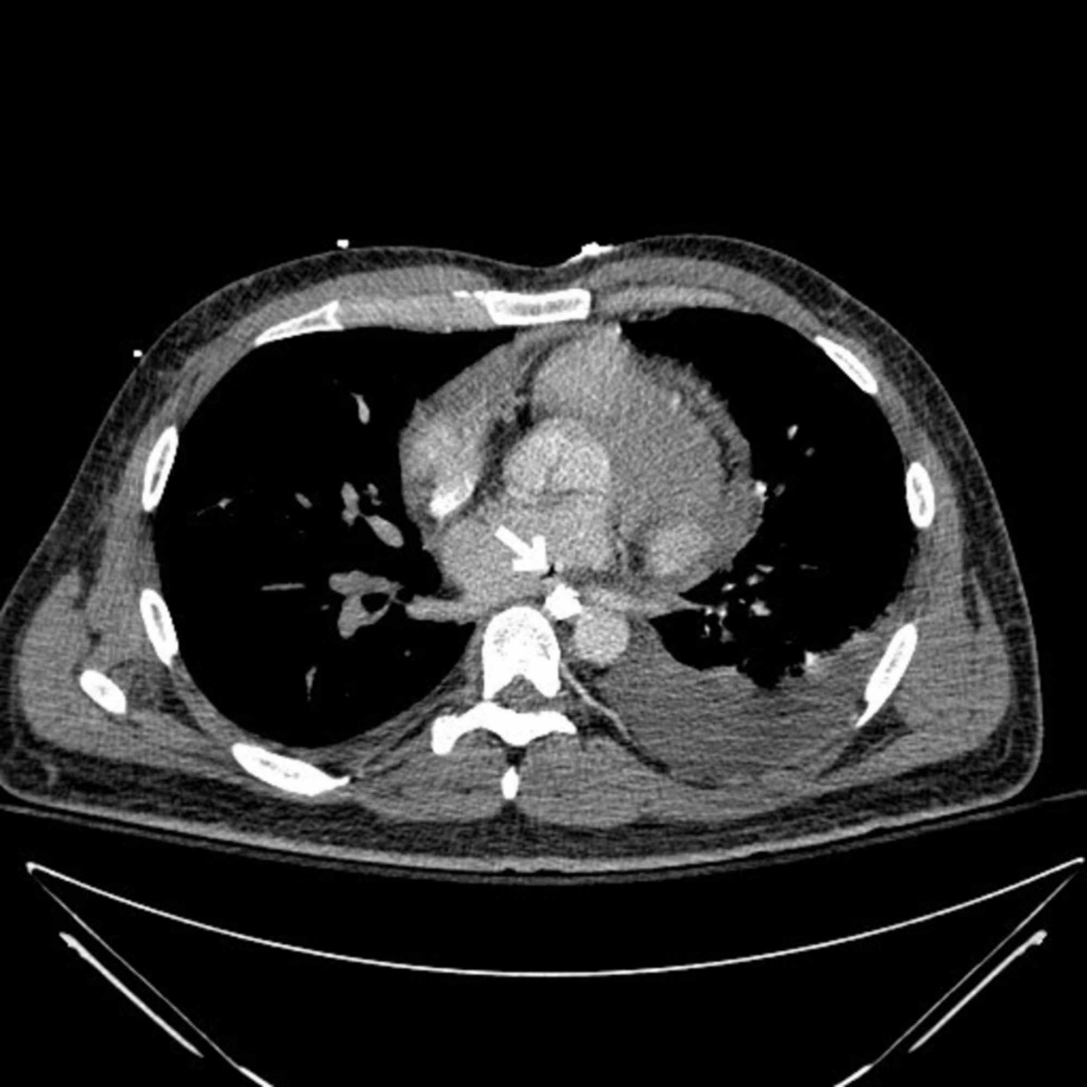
Chest CT (pulmonary veins) showing an atrial-esophageal fistula (arrowhead) CT: computed tomography
